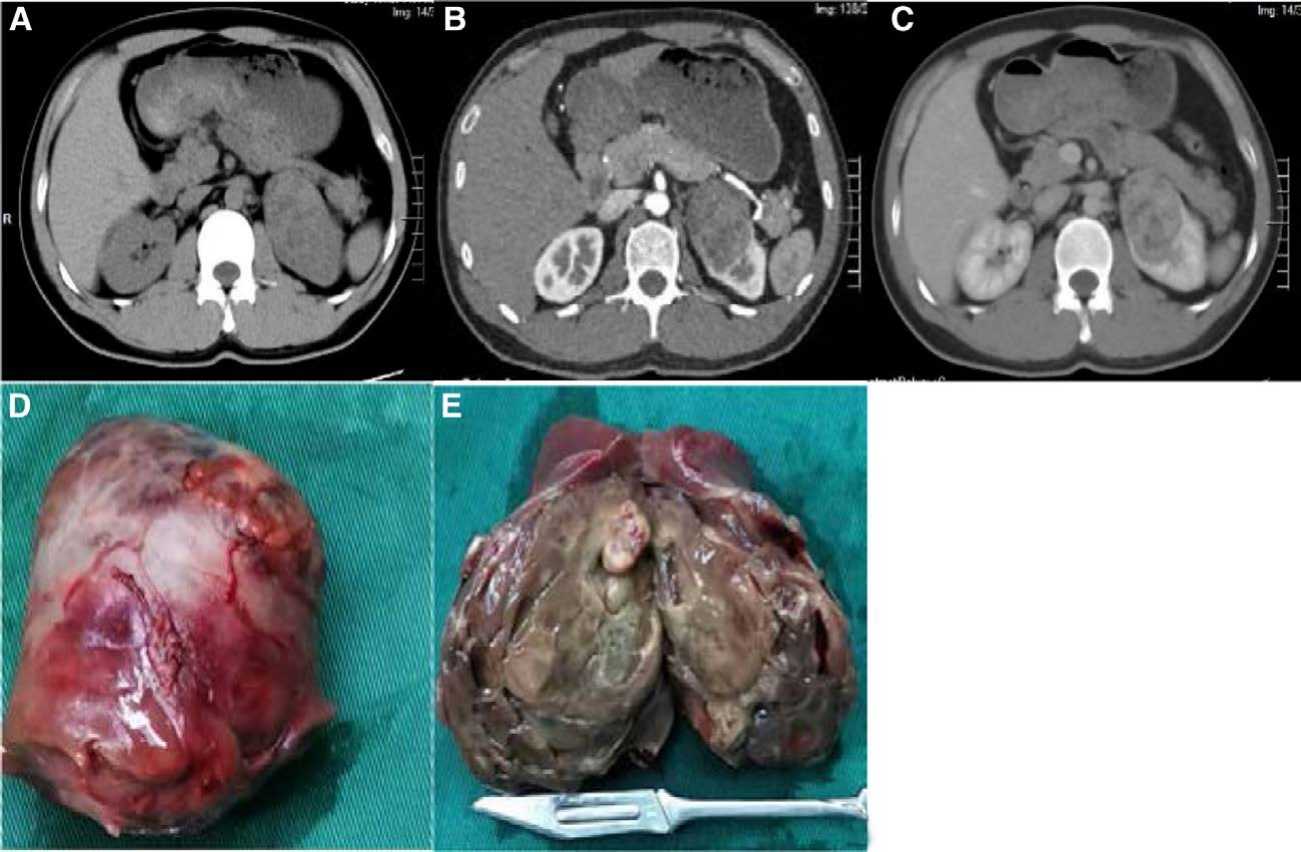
(A) Computed tomography (CT) showing a round mass in the upper left kidney on plain scan. (B and C) Contrast-enhanced CT showing uneven mild enhancement of the tumor, no abnormal density, and enhancement of the right kidney. (D and E) The internal tumor was grayish-yellow, grayish-red, soft, and cystic locally. CT = computed tomography.
